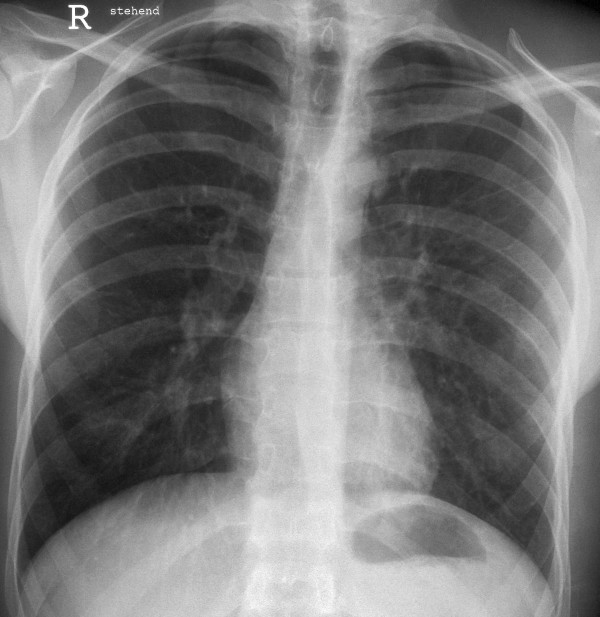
Chest radiography with reexpanded left lung and decreased pulmonary edema.
